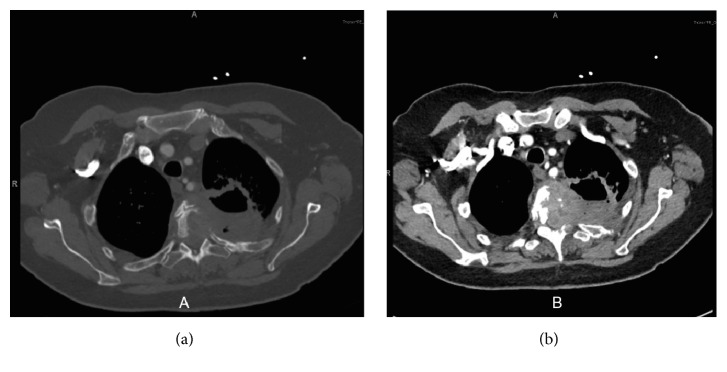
Computed tomography (CT) of the chest in the axial plane with (a) bone window and (b) soft tissue window.
